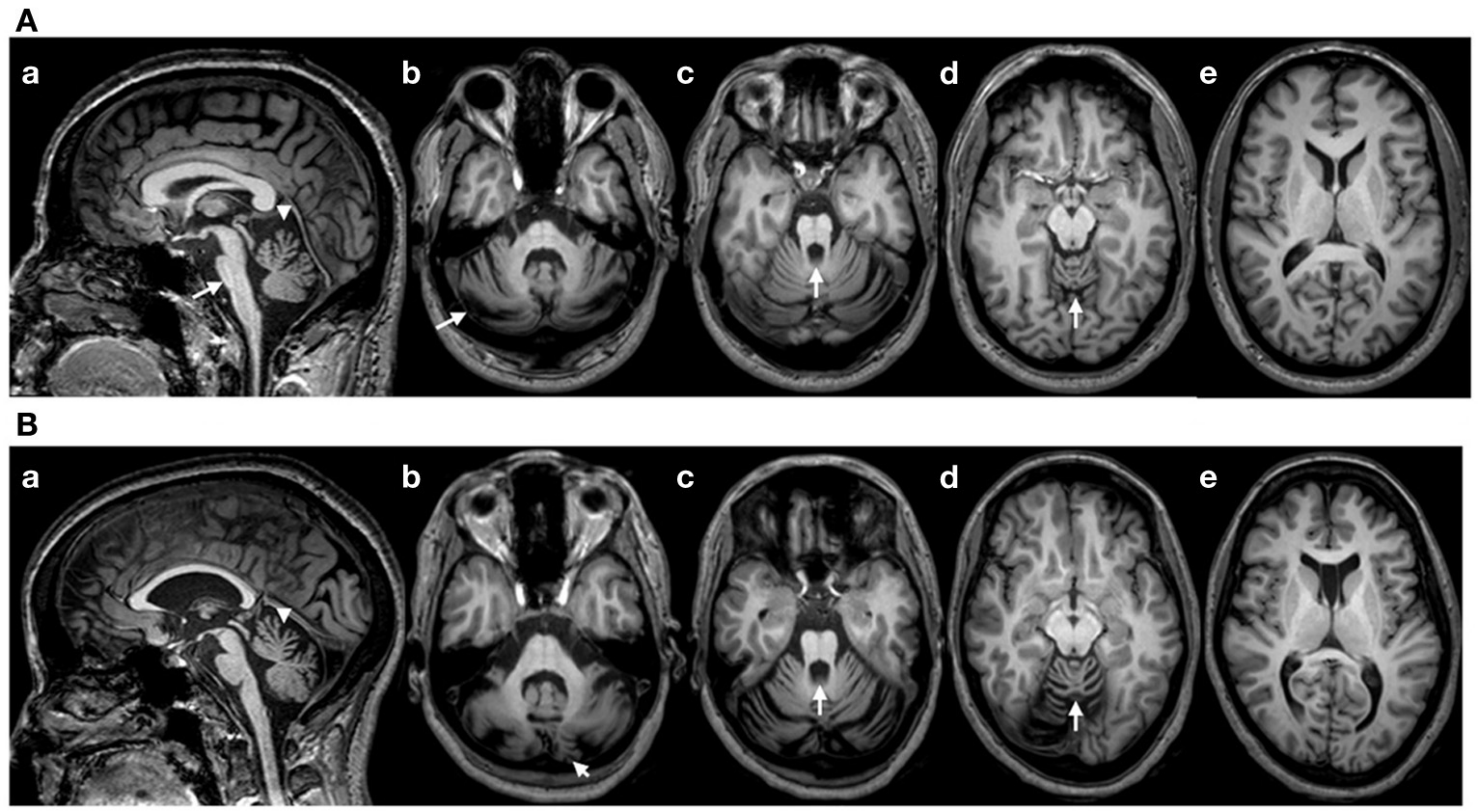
(A) Brain MRI (T1-weighted images) in patient 1. (a): sagittal image showing a flattening of the pons (arrow), atrophy of the upper vermis (arrowhead) and a thin spinal cord in upper cervical segments; (b–d): axial sections showing atrophy of the cerebellum at the level of the middle cerebellar peduncle (b; arrow: atrophy of the cerebellar cortex), the upper pons with enlargement of the 4th ventricle (c; arrow) and the upper vermis (d; arrow). (e): Normal appearance of the cerebral cortex (axial slice at the level of the head of caudate nucleus). (B) Brain MRI in patient 2 (T1-weighted images). Flattening of the pons is less severe as compared to patient 1 (a), whereas the degree of cerebellar atrophy is relatively similar (b–e). Note the presence of a cavum of the septum pellucidum in patient 2.
